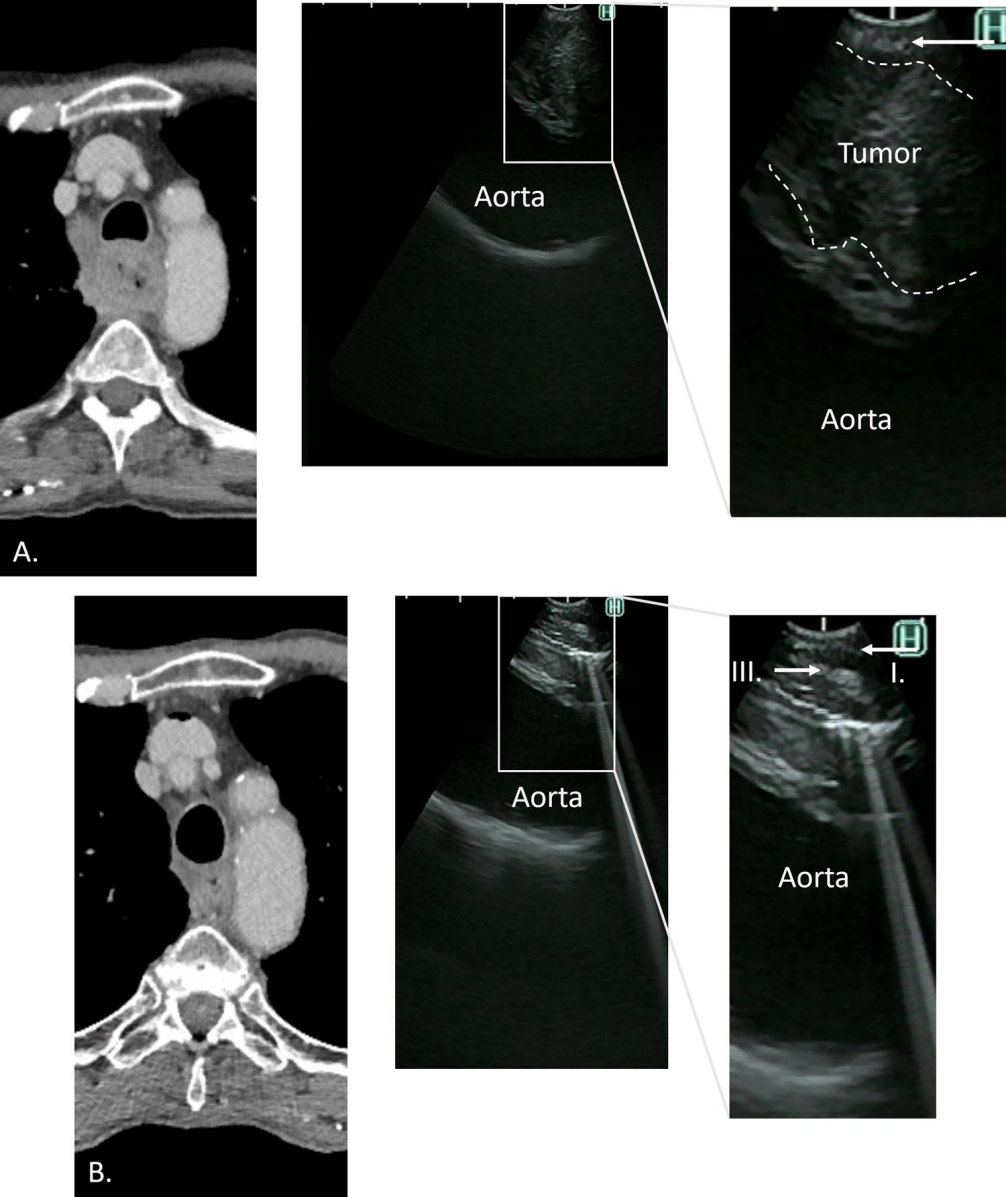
A A computed tomography CT (left) image of a patient with suspicion of tumor invasion into the tracheabronchial wall before dCRT. On the EBUS (right), the adventitia (third layer) is not recognizable, and tumor invasion was considered to be present. B A CT (left) and EBUS (right) image of the same patient after dCRT in which the third layer is recognizable on the EBUS, and tumor invasion was considered to be absent. EBUS, endobronchial ultrasonography
